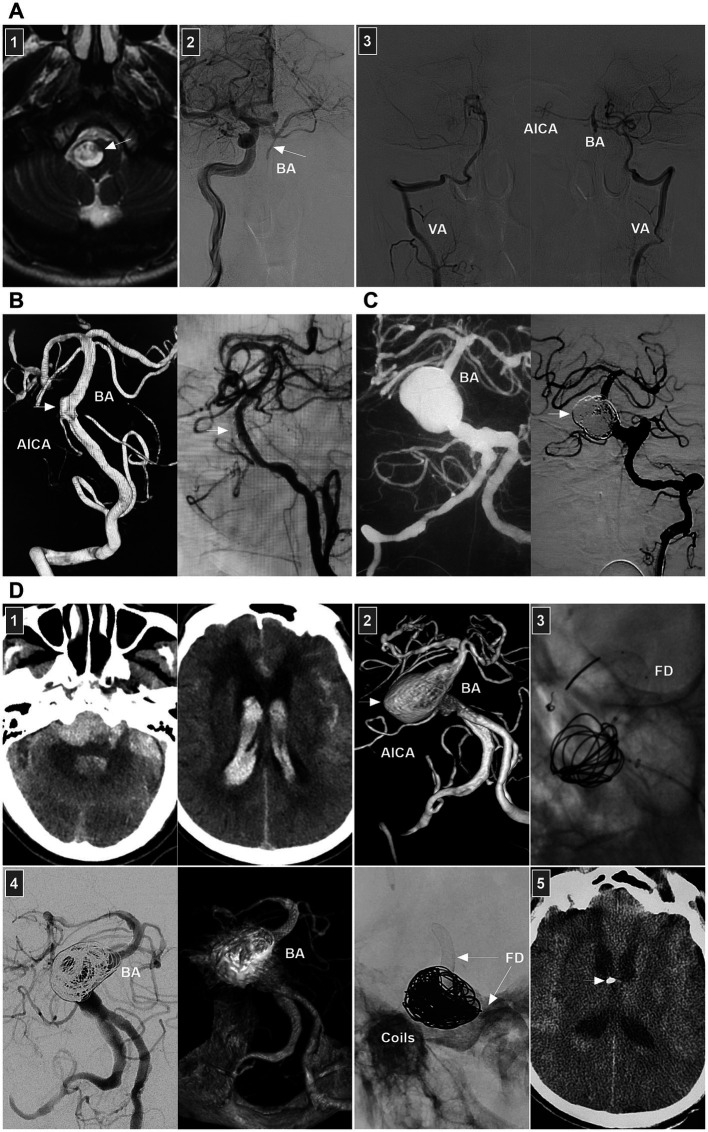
Treatment of BA dissections. (A) Panel 1: Magnetic resonance image showing a lesion (arrow) in front of brainstem. Panel 2: DSA showing the BA was occluded (arrow), the BA tip was supplied by posterior communicating artery. Panel 3: DSA images showing that bilateral VAs only supplied to the PICAs, lower BA and AICA. In the patient, the medication was given. (B) Left panel: DSA showing an unruptured BA dissection (arrow). Right panel: DSA showing delayed appearance of BA dissection after LEO stent deployment. (C) Left panel: Roadmap image showing a ruptured sidewall BA dissecting aneurysm. Right panel: DSA showing the aneurysm (arrow) was coiled under the assistance of traditional stent. (D) Panel 1: CT images (left and right panels) showing subarachnoid hemorrhage and intraventricular hemorrhage. Panel 2: DSA showing a giant BA dissecting aneurysm (arrow). Panel 3: X-ray image showing FD assisted coiling the aneurysm. Panel 4: DSA (left panel) and Vaso-reconstructive image (right panel) showing that the aneurysm was embolized. Panel 5: X-ray image showing the FD (arrows) and coils. Panel 6: Postoperative CT showing the external ventricular drainage (arrow) was performed due to acute hydrocephalus. AICA, anterior inferior cerebellar artery; BA, basilar artery; CT, computed tomography; DSA, digital subtraction angiography; EVT, endovascular treatment; FD, flow diverter; PICA, posterior inferior cerebellar artery; VA, vertebral artery.
